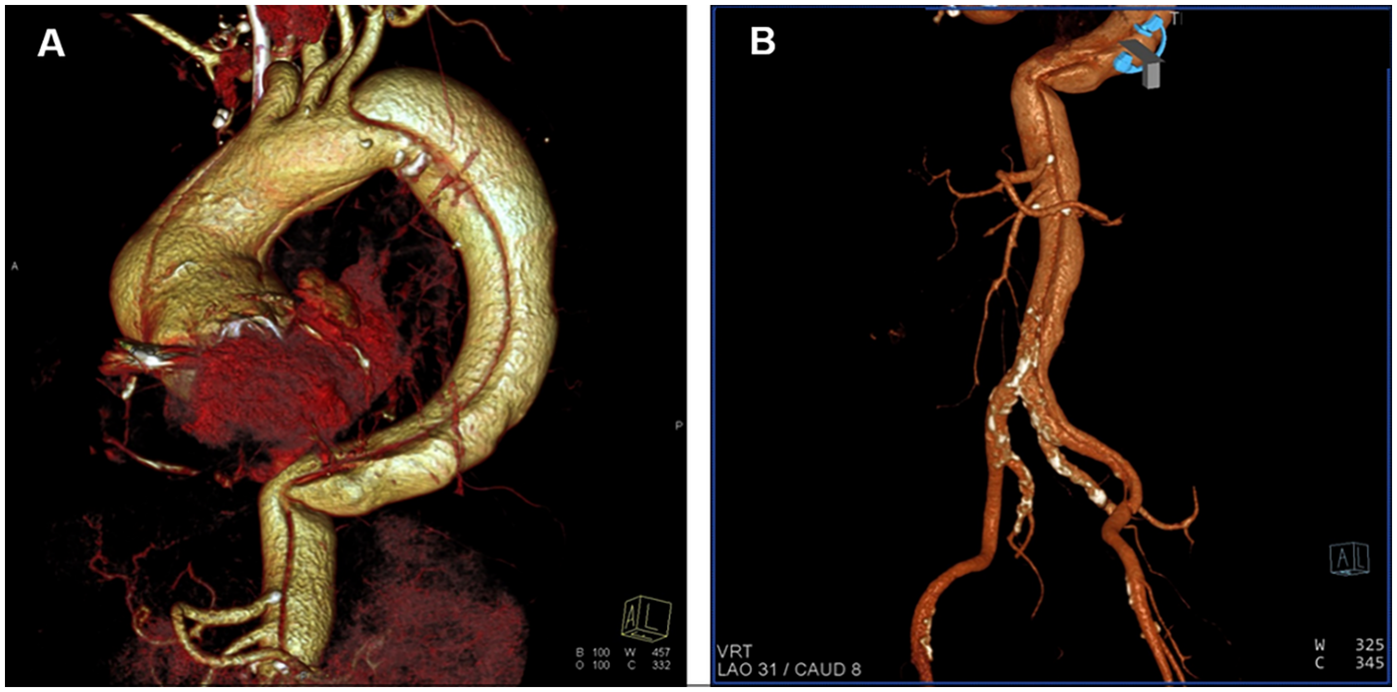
Three-dimensional (3D) reconstruction images from computed tomography angiography. (A) Thoracic reconstruction showing dissection of the ascending aorta, the aortic arch, and the descending aorta. (B) Abdominal reconstruction showing dissection of the abdominal aorta, the left common iliac artery and the femoral artery.
